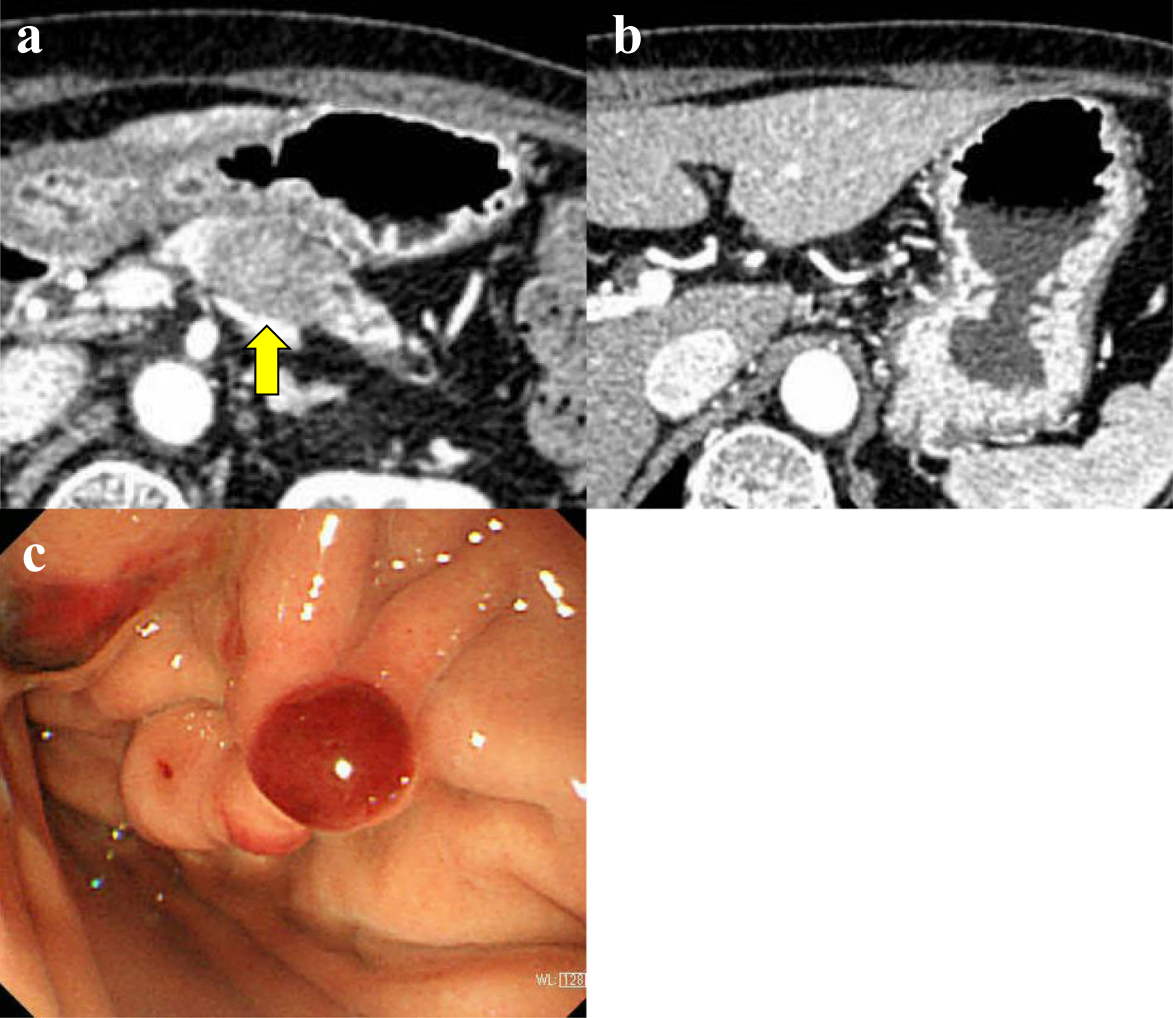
Images prior to the initial operation for pancreatic cancer. a Computed tomography revealed a low-density mass in the body of the pancreas (arrow). b Lymph node swelling around the stomach before surgery was not observed. c The puncture site of fine needle aspiration
